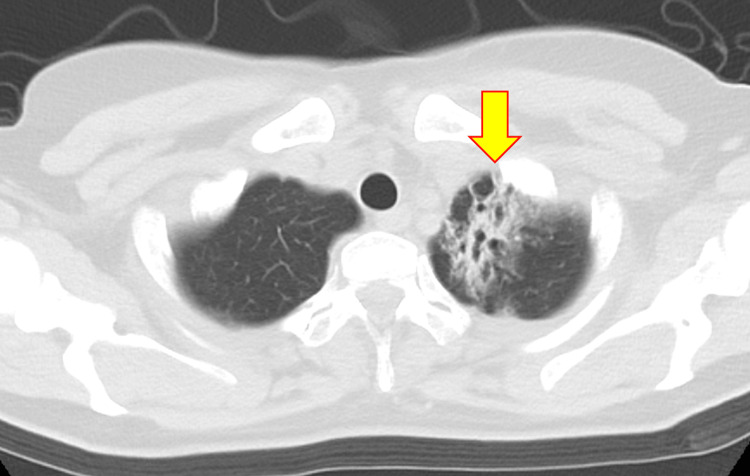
Chest CT scan showing postradiation changes in the left lung apex and upper lobe (yellow arrow).
